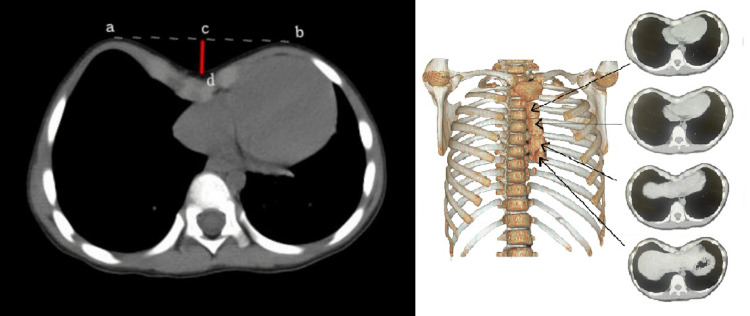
Selection of critical CT slices for severe pectus excavatum assessment. Left panel: How was the image of interest in a set of cross-sectional series selected. ab: a tangent line connecting the highest point of the front chest on both sides. cd: a perpendicular segment from the deepest point of the concave to line ab. Right panel: different levels of axial images were chosen for model building. Note only four levels of images are shown.
